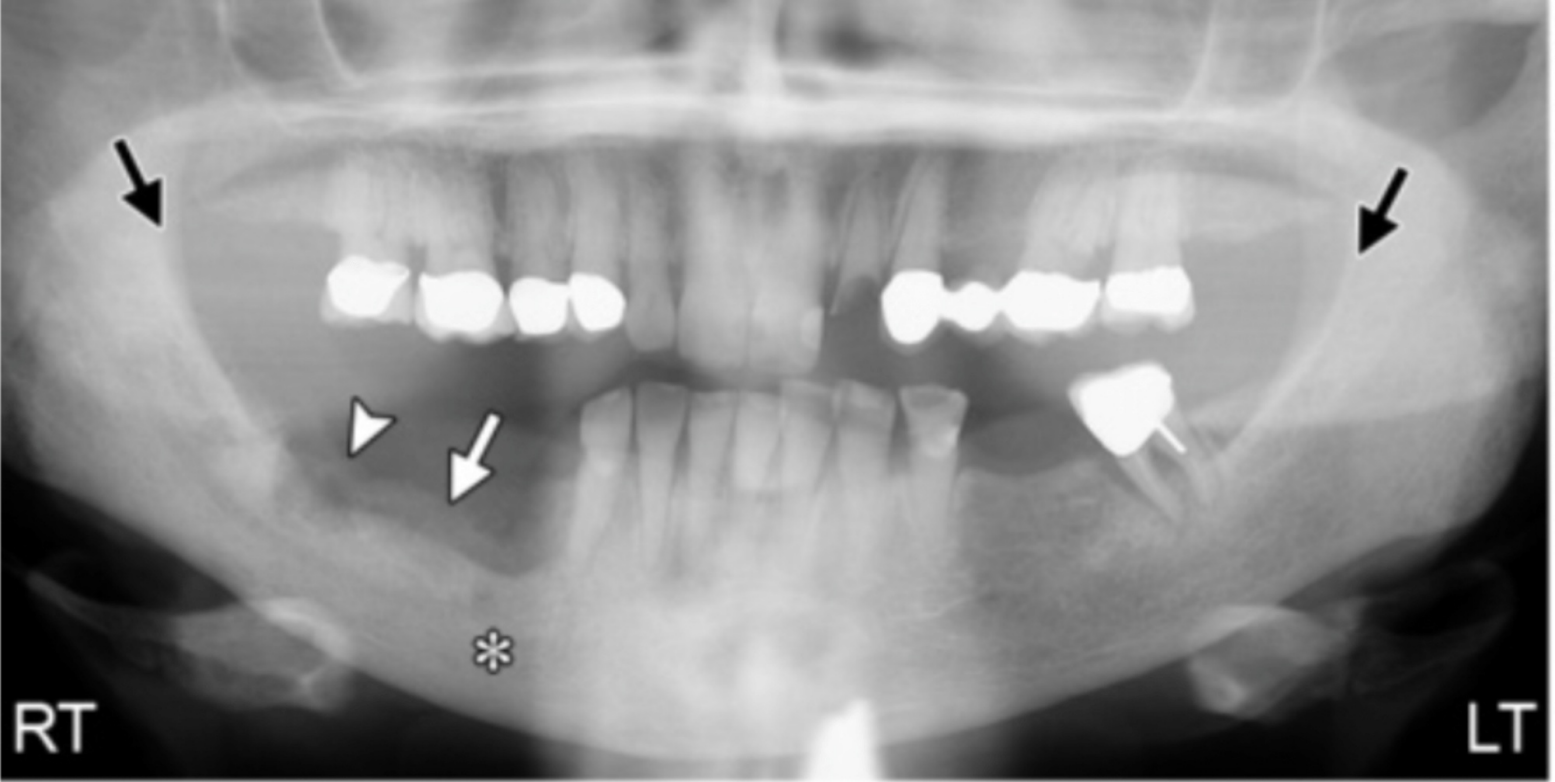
The appearance of ONJ on panoramic radiograph Panoramic radiograph view of ONJ shows alveolar bone reaction at the area of the empty socket of previously extracted teeth (arrowhead and white arrow), and loss of cortication on the right side compared to the left (black arrows) *Sclerosis ONJ: osteonecrosis of the jaw
